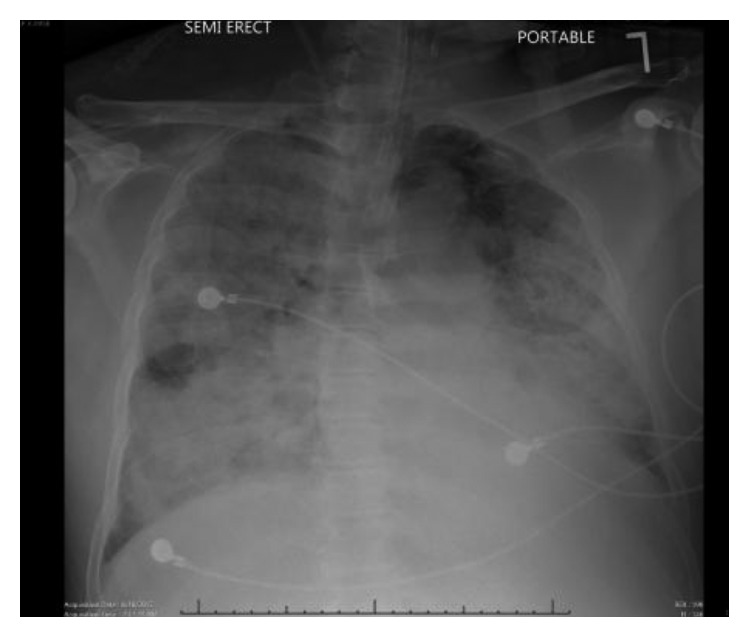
Chest X-ray showing diffuse bilateral alveolar infiltrates.
